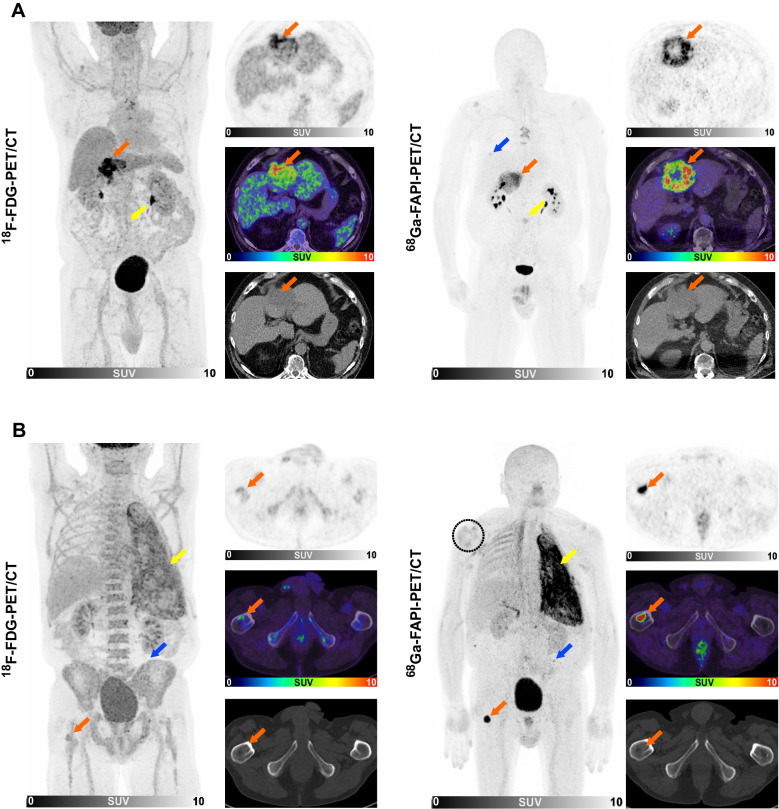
Representative images of ^18^Fluor-fluorodeoxyglucose- (^18^F-FDG) and ^68^Gallium-labelled-fibroblast activation protein inhibitor- (FAPI) positron emission tomography with computed tomography (^68^Ga-FAPI-PET/CT) in 2 patients with oligo-metastatic extra-cervical CUP. A 76 years old patient with an intrahepatic metastasis (red arrow) detectable in both, ^18^F-FDG- and ^68^Ga-FAPI-PET/CT, but more clearly delineated in the ^68^Ga-FAPI-PET/CT scan due to higher contrast with the liver background. ^68^Ga-FAPI-PET/CT shows additionally a lesion above the dome of the liver consistent with peritoneal carcinomatosis (blue arrow) and a bone lesion in the vertebra (yellow arrow), which cannot be clearly distinguished in ^18^F-FDG-PET/CT. B 77 years old patient with pleural carcinomatosis on the left side (yellow arrow) and a bone metastasis in the proximal right femur (red arrow) which is better distinguishable in ^68^Ga-FAPI- compared to ^18^F-FDG-PET/CT due to better tumor-to-tissue contrast. In the left ilium, ^68^Ga-FAPI-PET/CT also shows a second bone metastasis (blue arrow), which cannot be clearly delineated in ^18^F-FDG-PET/CT due to the bone marrow activation (displayed in Fig. 3). As a secondary finding, the right shoulder joint appears more intensely in the ^68^Ga-FAPI-PET/CT scan (dashed black circle)
